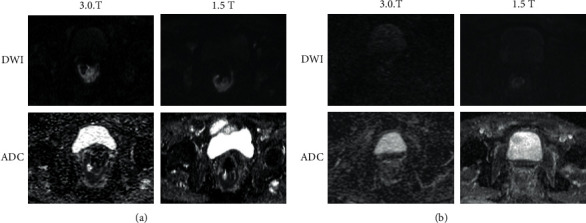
The picture shows DWI and ADC maps in CR at both field strength pre-CRT (a) and post-CRT (b). In post-CRT, on 3.0 T, the fibrotic tissue is better emphasized with no restriction of diffusion compared to 1.5 T where the difference with pre-CRT is less highlighted. In addition, on DWI, post-CRT at 1.5 T is more evident because of the shine through artifact of the rectal wall that could be misled as persistent viable tumor, while on 3.0 T is less evident despite the higher noisiness of the image.
